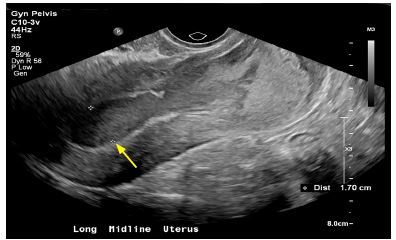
Formal pelvic ultrasound showing mixed echogenic contents contained within the uterus (arrow).
